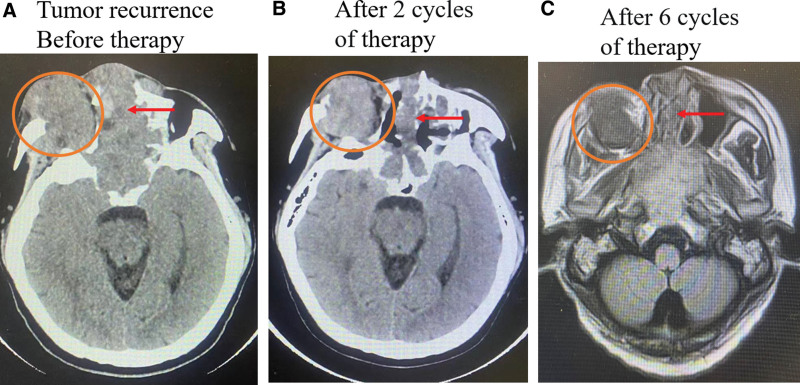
Representative imaging assessments during each period. Head computed tomography (CT) assessment before therapy (A) and after 2 cycles of therapy (B). Head magnetic resonance (T2-FLATR) assessment after 6 cycles of therapy (C). Red arrows indicate the nasal cavity tumor. The orange circles indicate the right orbital tumor.
